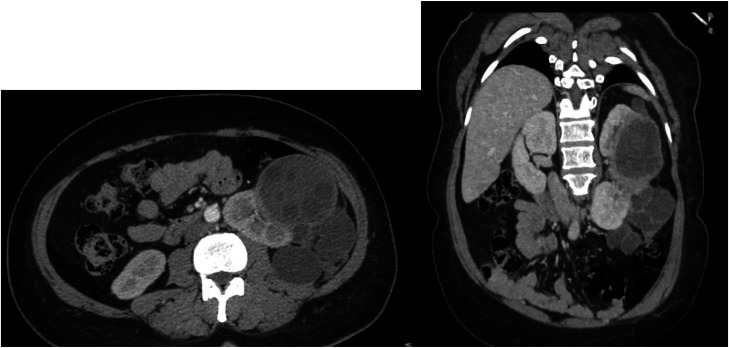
Portal venous phase CT findings (axial and coronal sections): huge cystic lesion arising from the left renal cortex, located anteromedially with extension to both poles. The lesion demonstrated: Multiple daughter cysts, irregular wall thickening, a cortical defect at the inferior pole with localized rupture of several daughter vesicles into the perirenal space.
